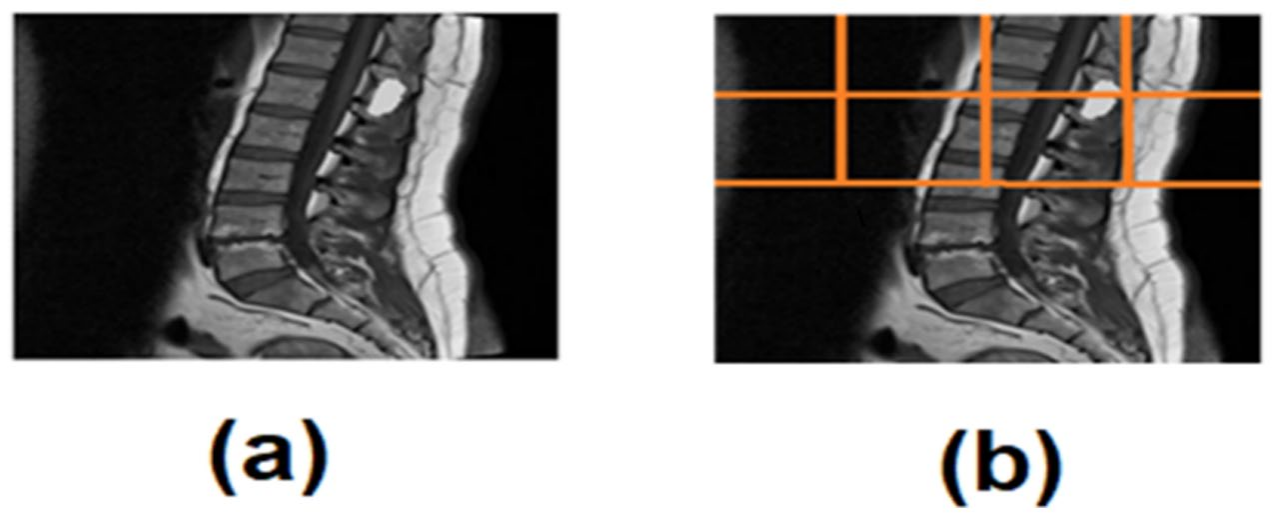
(a) Spinal tumor MRI, (b) ROI quadrant analysis of spinal tumor MRI.
